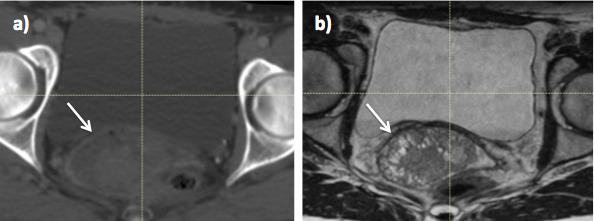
Radiotherapy planning imaging in Stage 2B cervix cancer (a) CT and (b) MRI. On MRI, the cervix tumour (arrow) is easily differentiated from normal bladder and rectum, which is not possible on CT.
